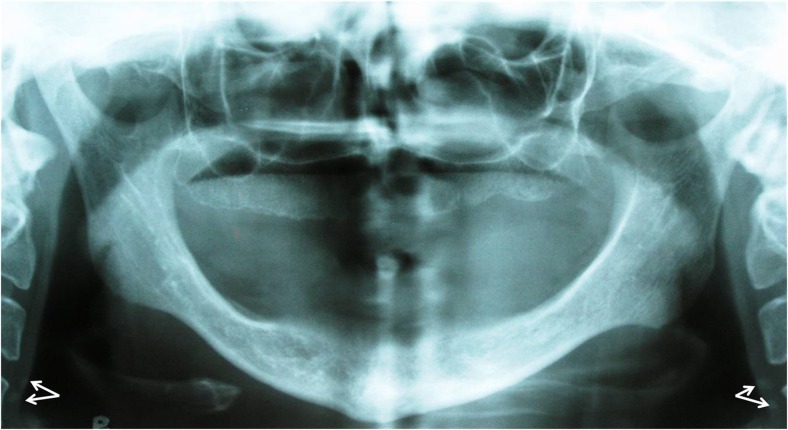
Patients were informed of the observations in their panoramic radiography. In case of consent, they were sent for DS.
